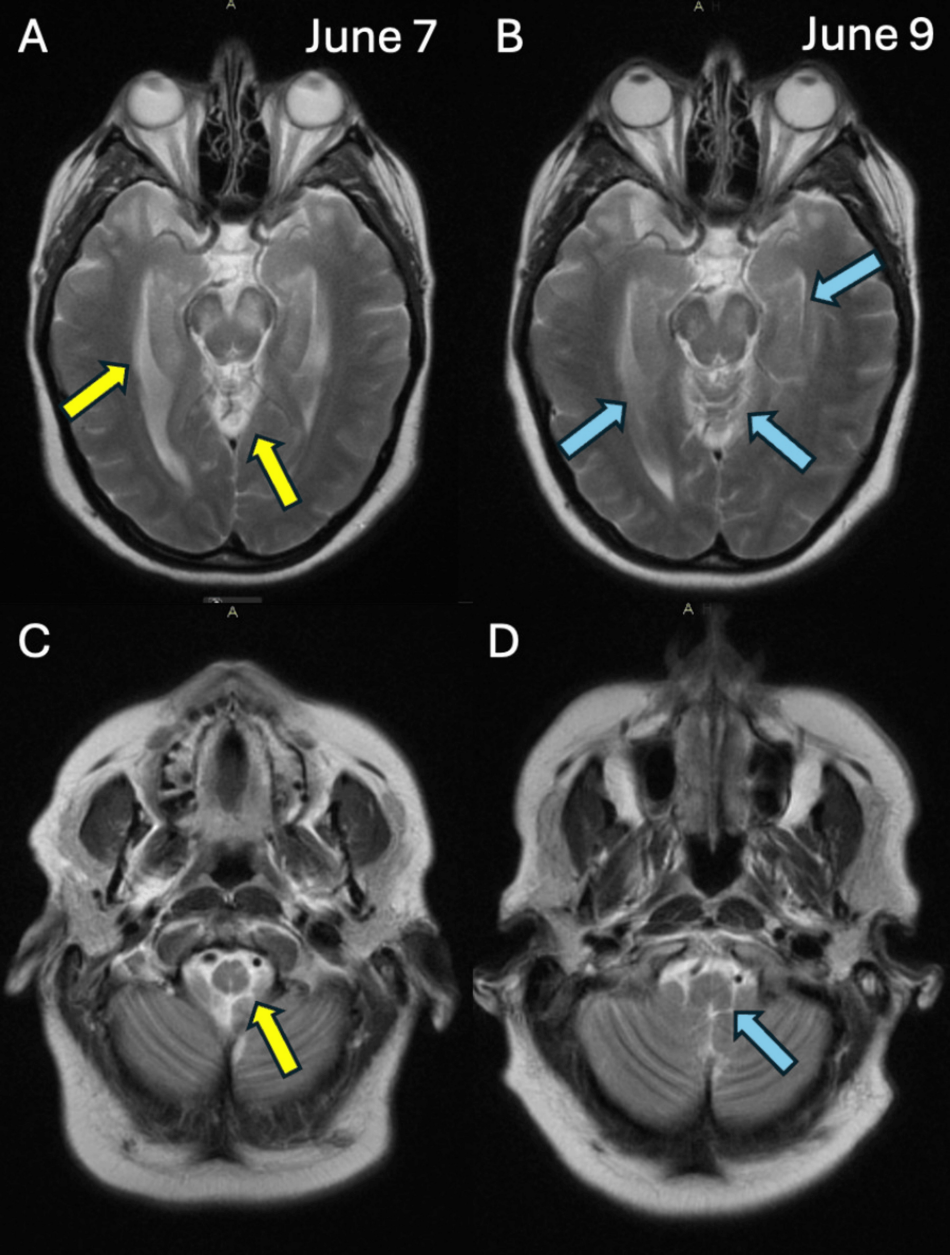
Head MRI edema changes from 6/7 to 6/9 Note: Panels A and C (June 7) show hippocampal and brainstem edema (yellow arrows). Panels B and D (June 9) show improvements in edema (blue arrows).
